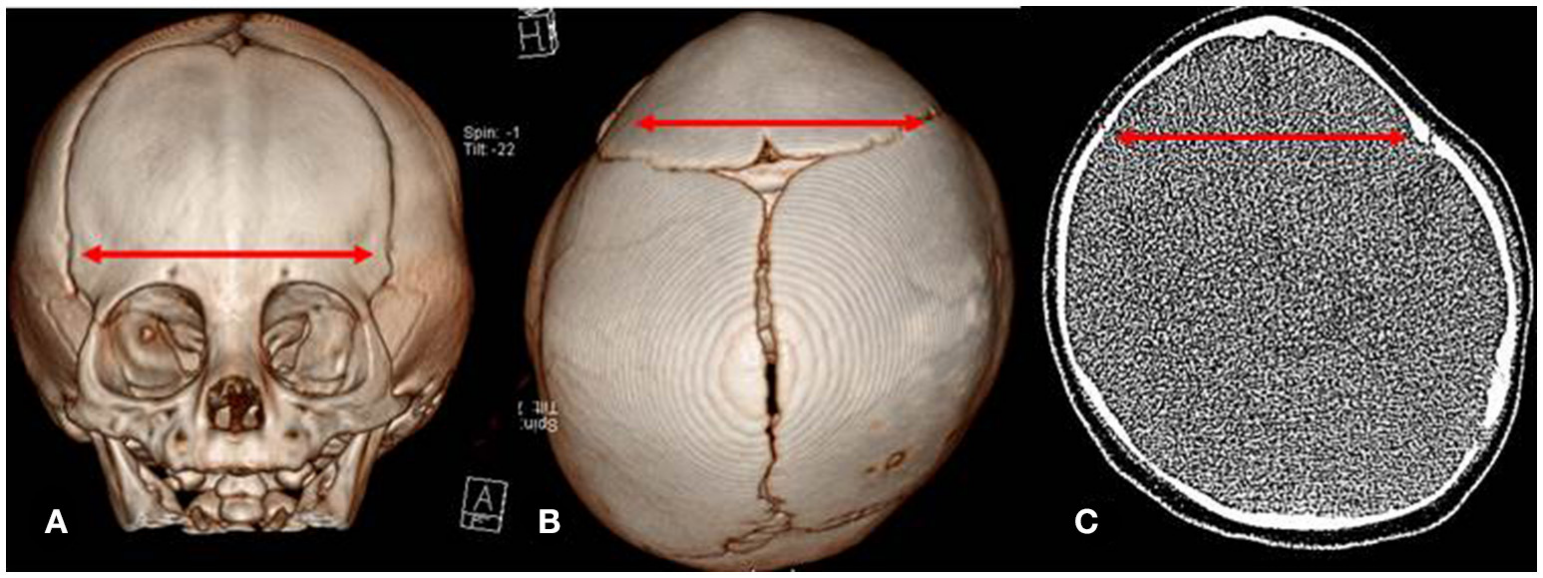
Computed Tomography (CT) images of metopic craniosynostois. (A) Anterior view of metopic deformity (B) Vertex view of metopic deformity with mild occipital deformational plagiocephaly (C) Axial view of metopic deformity. *Red arrow* indicates bifrontotemporoparietal narrowing in each view.
